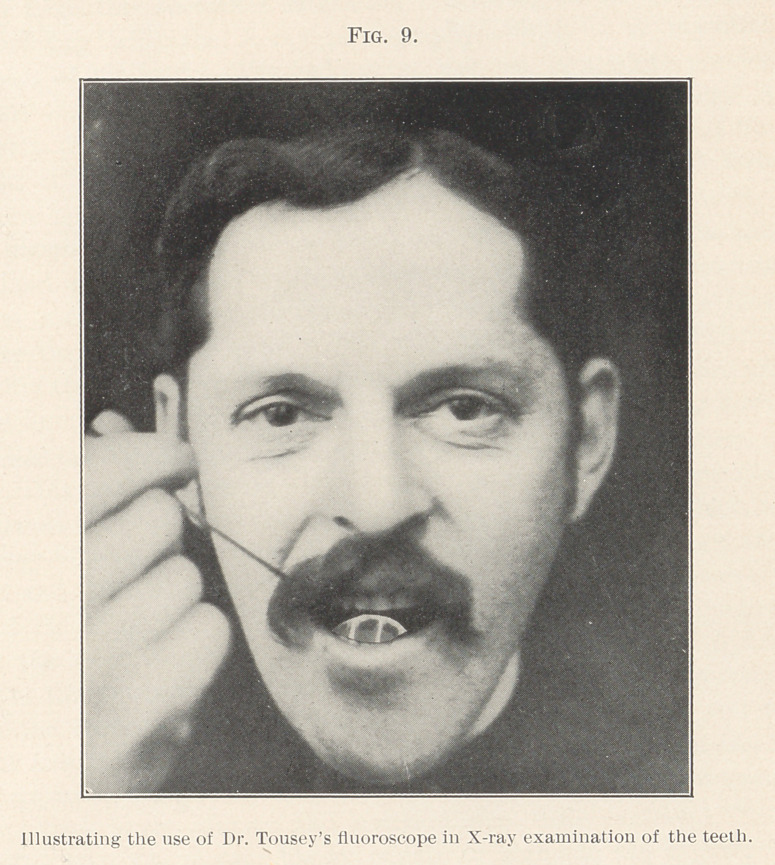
# Radiotherapy in Pyorrhœa Alveolaris, and Dental Radiography1Read before The New York Institute of Stomatology, March 1, 1904.
